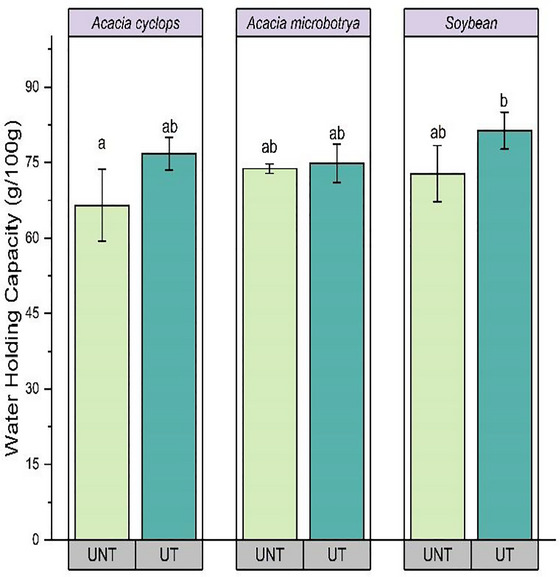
Change in water‐holding capacity *Acacia cyclops*, *Acacia microbotrya*, and soybean for untreated and ultrasound‐treated conditions. UNT, untreated; UT, ultrasound‐treated (80 W, 20 kHz, 20 min: highest yield condition).
